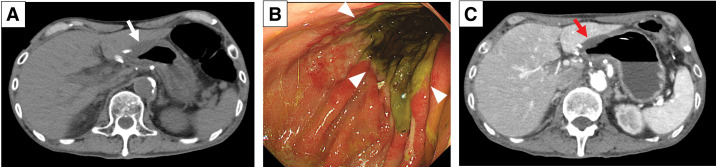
Postoperative CT images and endoscopic findings. (A) Marked edematous changes (white arrow) of the remnant stomach wall (POD 3). (B) Circumferential mucosal necrosis (arrowheads) of the distal remnant stomach (POD 8). (C) Poor enhancement (red arrow) of the distal remnant stomach (POD 14).
